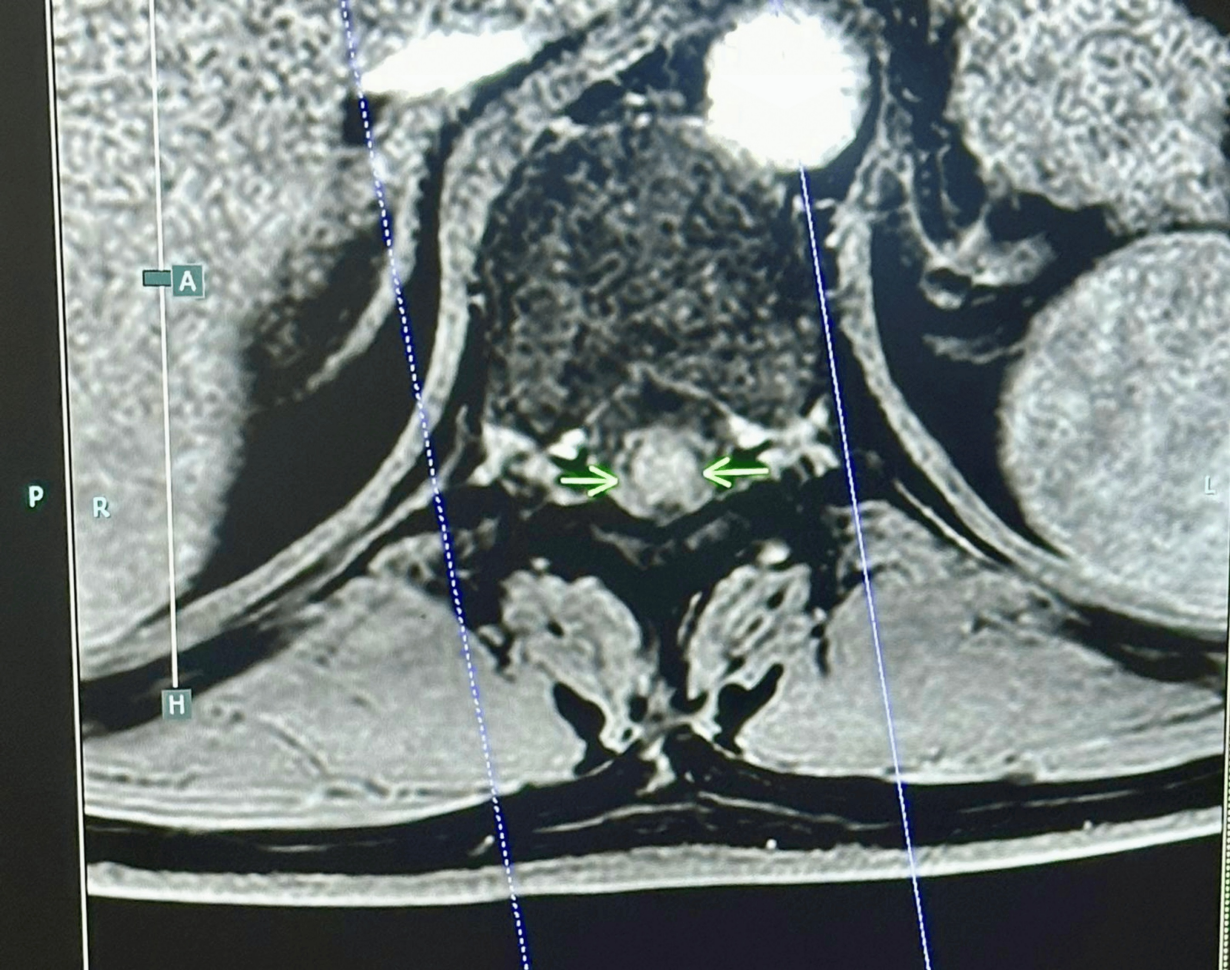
MRI of the spine Focal T2 hyperintense lesion at T10-T11 with patchy gadolinium enhancement involving the thoracic spinal cord, consistent with inflammatory myelitis.
